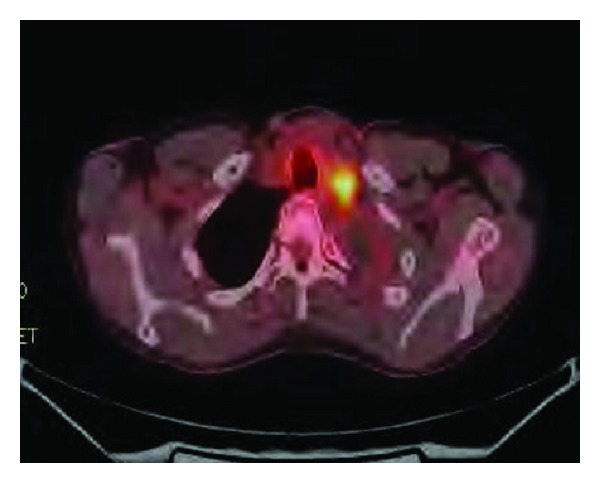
Fused fluorodeoxyglucose (FDG) positron emission tomography (PET) CT shows hypermetabolic left supraclavicular lymphadenopathy (yellow).
